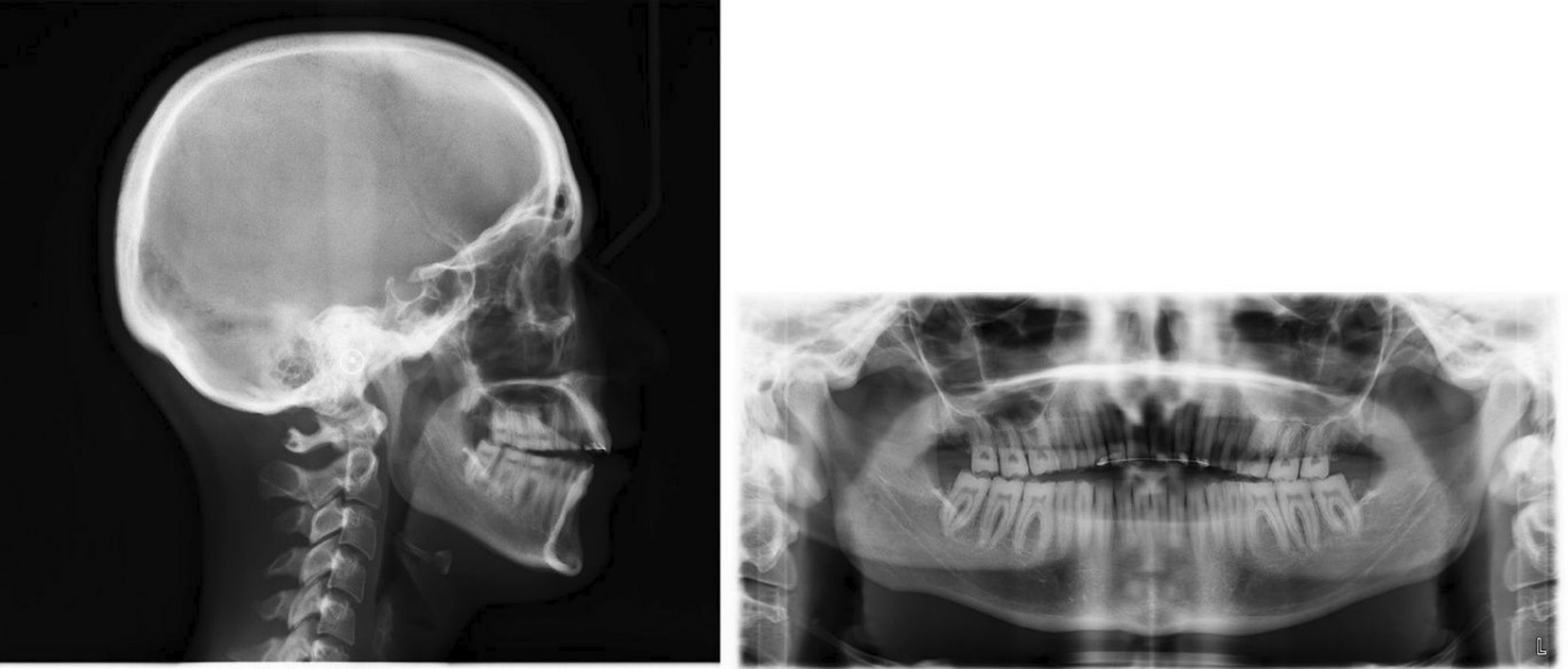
Pre‐treatment radiographs
